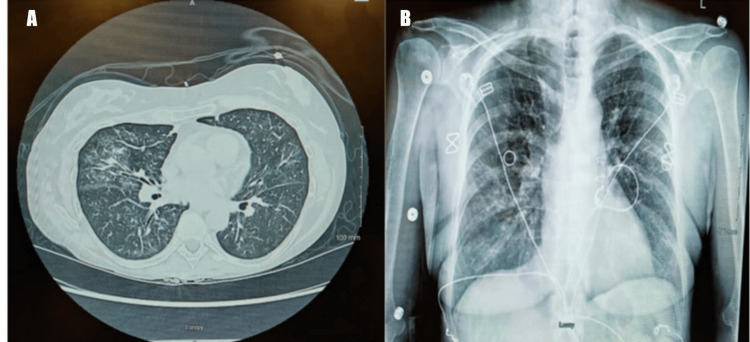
Pulmonary manifestations of anti-MPO-positive GPA: bilateral multifocal ground-glass opacities, tree-in-bud nodules, and patchy alveolar infiltrates demonstrating active vasculitic involvement on CT scan (a) and X-ray (b) GPA, granulomatosis with polyangiitis; MPO, myeloperoxidase
